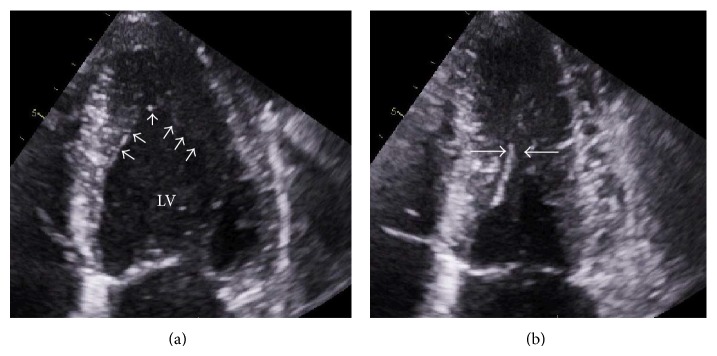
Apical hypertrophic cardiomyopathy. Transthoracic echocardiogram showing left ventricular (LV) apical hypertrophy in diastole ((a), arrows) and midcavity obliteration at end-systole ((b), arrows).
